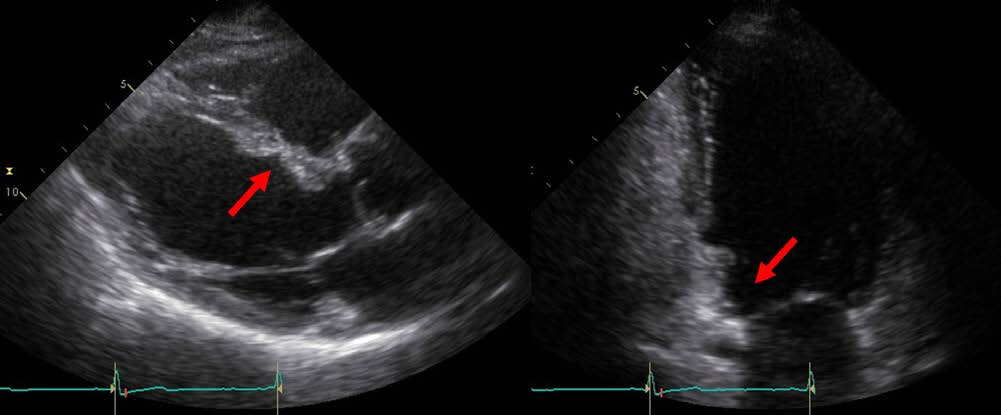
Ultrasound echocardiographic images. Ultrasound echocardiography revealed thinning of the midanteroseptal (left) and basal inferior (right) walls (arrow).
